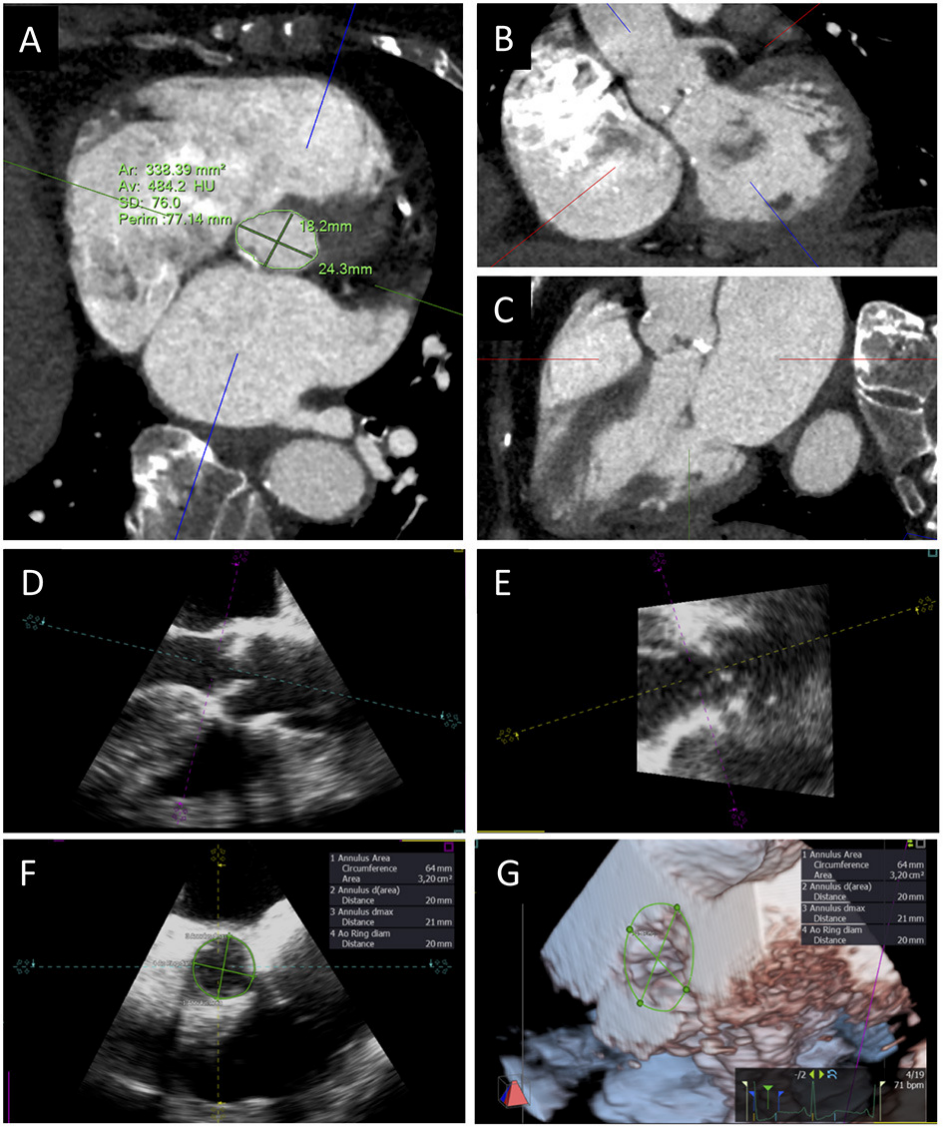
Aortic annulus sizing with CT and 3D TEE. Transverse CT plane aligned at the lowest insertion points of aortic leaflets (A) and orthogonal planes oriented along the main axis of LVOT in short (B) and long axis (C) views. TEE long-axis mid-esophageal view (D), view of the aortic annulus perpendicular to the mid-esophageal view (E), aortic annulus sizing (F), and 3D visualization and measurement of aortic annulus (G).
